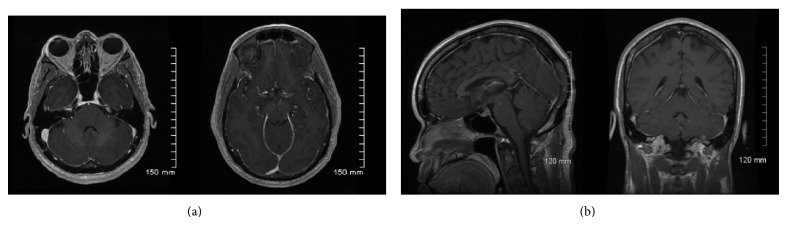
Follow-up MRI: T1 postcontrast axial images on the top row, sagittal and coronal on bottom row.
